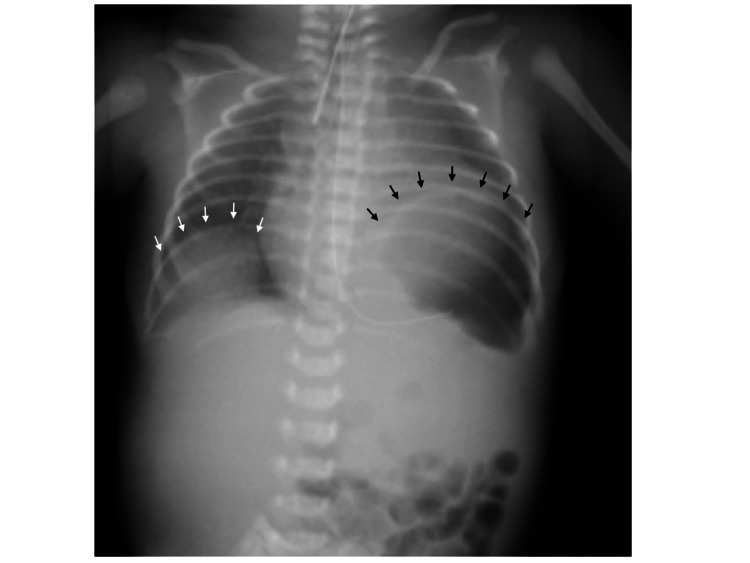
Preoperative chest X-ray Preoperative anteroposterior X-ray shows bowel gas in the left thorax (black arrows) and partial elevation of the diaphragm in the right thorax (white arrows).
